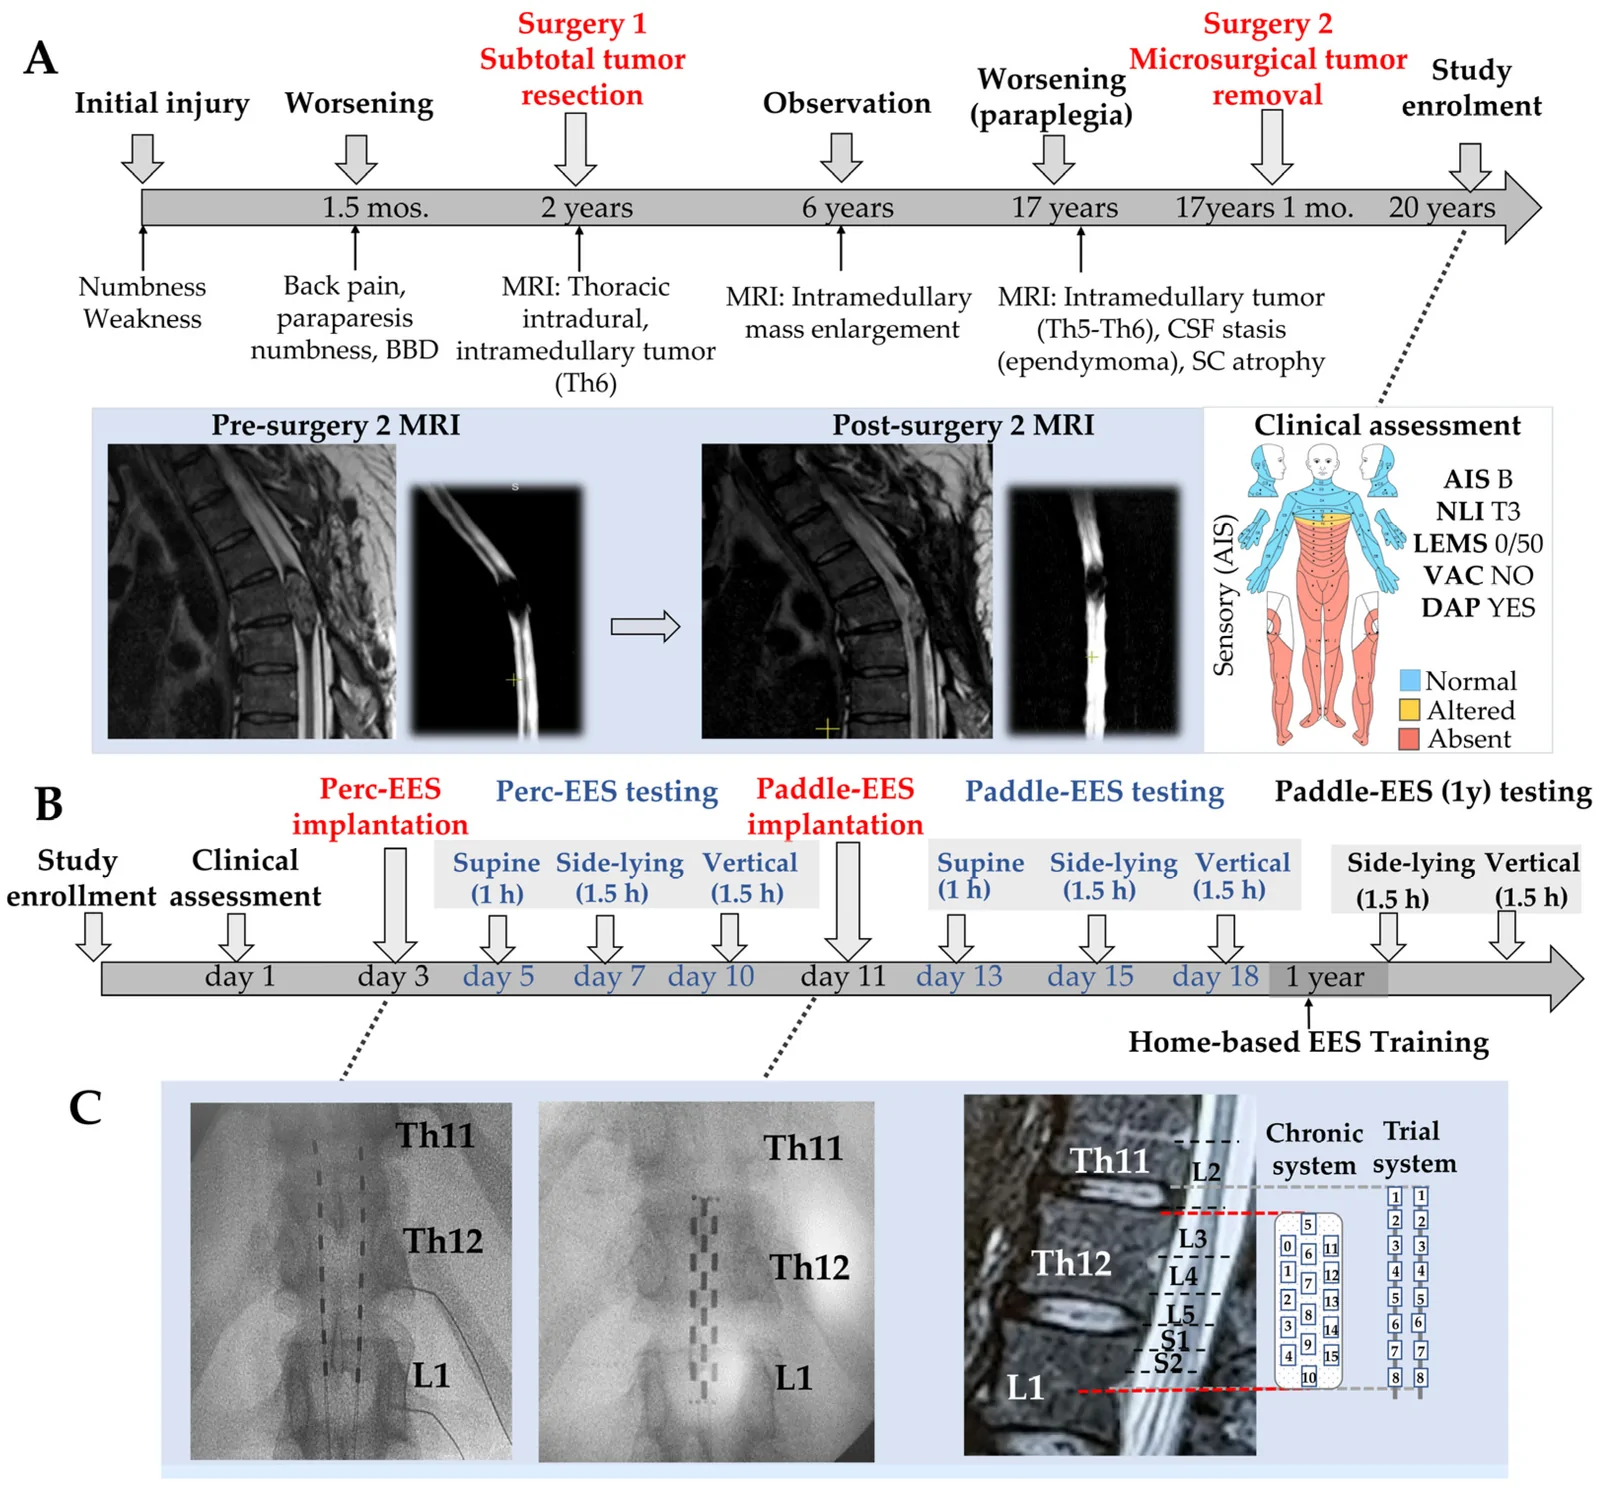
Clinical history and study timeline. (A) Overview of the patient’s medical history. Key interventions include: prednisolone pulse therapy and two surgical procedures: surgery 1 (subtotal tumor resection, two years after symptoms onset) and surgery 2 (microsurgical tumor removal, 2016). MRI findings (right panels) show the spinal cord at the Th5–Th6 segment. The pre-surgery MRI (17 years after symptoms onset and symptoms worsening: paraplegia) and post-surgery MRI (18 years after symptoms onset) demonstrate stable atrophic changes in the spinal cord without signs of progressive growth. (B) Study timeline and experimental design. Clinical assessment included the ISNCSCI protocol and questionnaires (quality of life, mental health, and bowel, bladder, and sexual functions). Electrophysiological testing with EMG registration during voluntary movement attempts and epidural electrical stimulation (EES), including Pecr-EES, Paddle-EES testing and Paddle-EES (1y) testing phases, enabled training and assessment in three positions: supine, side-lying (with the top leg suspended), and upright (in a body weight support system). (C) Electrode localization. X-ray images showing the percutaneous lead position during trial (Perc-EES) (implanted at the Th11–L1 level) and the permanent paddle electrode array (Paddle-EES) (Medtronic 5-6-5) lead position. The right panel displays a zoomed CT scan of the lumbar spinal cord with corresponding spinal segments (L2–S1) and the correlated electrode position relative to the lumbosacral locomotor circuitry. The red color on the timeline indicates electrode implantation and surgical procedures. BBD—bladder and bowel disorder; MRI—magnetic resonance imaging; CSF—cerebrospinal fluid; ISNCSCI—International Standards for Neurological Classification of Spinal Cord Injury; EMG—electromyography; EES—epidural electrical stimulation; CT—computed tomography: AIS—ASIA Impairment Scale; NLI—neurological level of injury; LEMS—lower extremity motor score; VAC—voluntary anal contraction; DAP—deep anal pressure.
